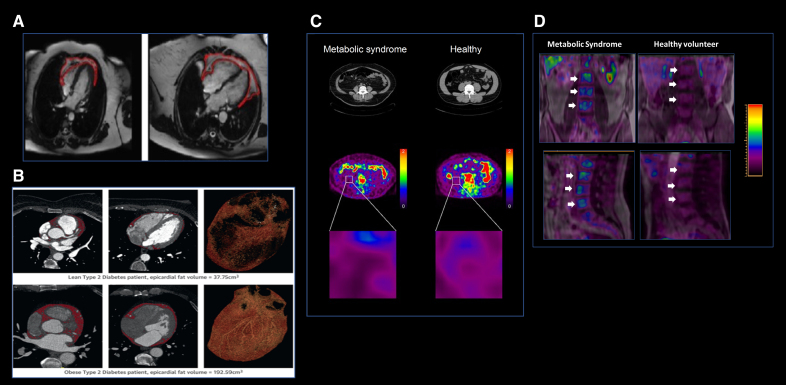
Adipose tissue and bone marrow imaging. A, Example of magnetic resonance (MR) images showing automated periadipose tissue segmentation and predicted segmentation quality. Adapted from Ardissino et al^104^ with permission. B, Cardiac computed tomography (CT) images of epicardial fat segmentation from a patient with diabetes. The lower row shows increased epicardial fat volume in a patient with obesity and diabetes compared with the lean patient in the upper row. Adapted from Levelt et al^144^ with permission. C, ^18^F-FDG (^18^F-fluorodeoxyglucose) positron emission tomography (PET)/CT images of visceral adipose tissue (VAT) and subcutaneous adipose tissue in a patient with metabolic syndrome (left) and a healthy individual (right). ^18^F-FDG uptake in VAT is notably higher in patients with metabolic syndrome compared with healthy individuals. Adapted from Pahk et al^103^ with permission. D, ^18^F-FDG PET/MR imaging of the bone marrow in metabolic syndrome and healthy individuals. The upper figure demonstrates increased ^18^F-FDG uptake in the lumbar vertebrae (white arrows) of a patient with metabolic syndrome, whereas the lower figure shows lower uptake in a healthy individual. Images were obtained from the PESA study (Progression of Early Subclinical Atherosclerosis).
